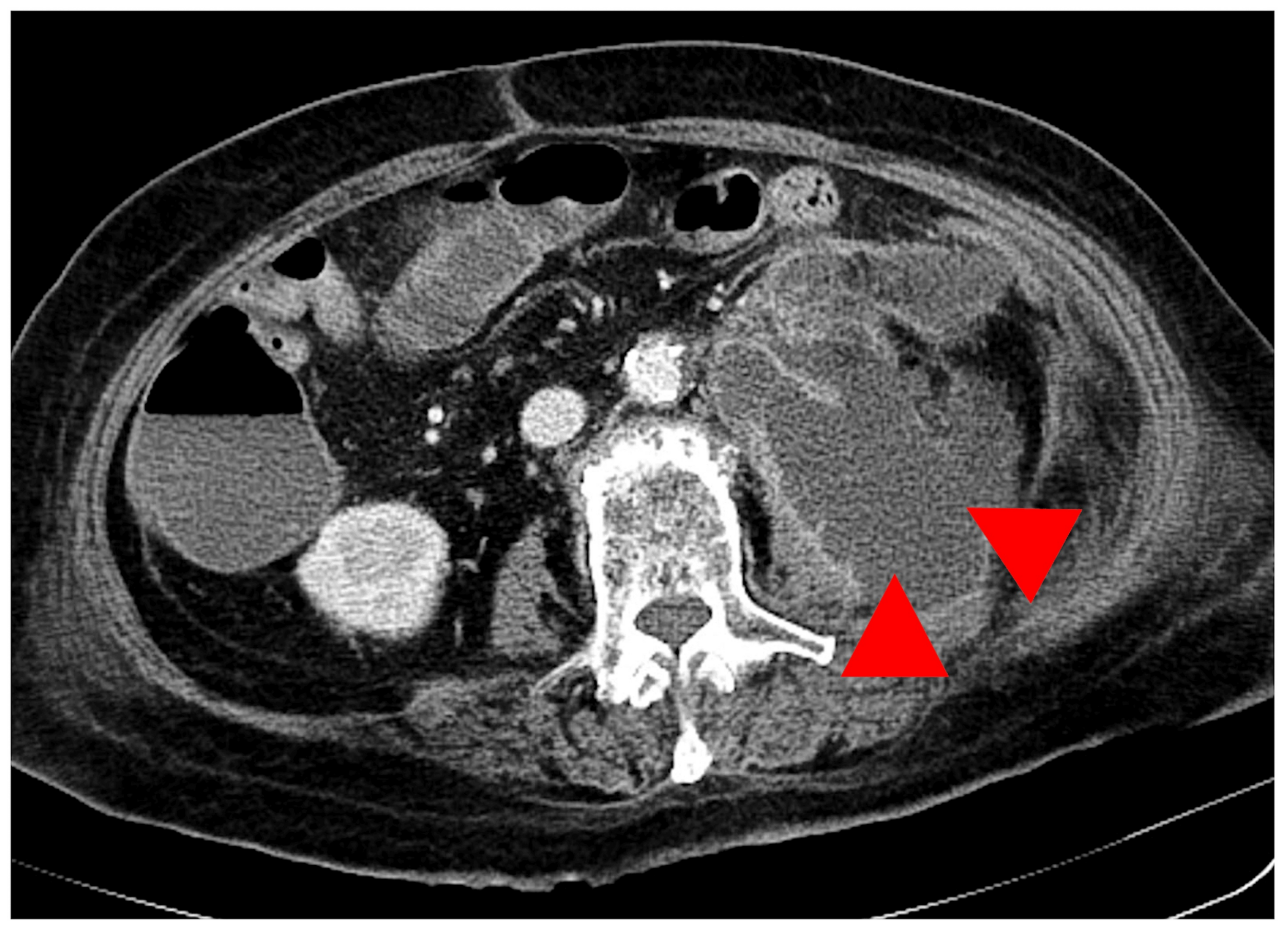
CT scan revealed a retroperitoneal abscess (red arrowheads).
